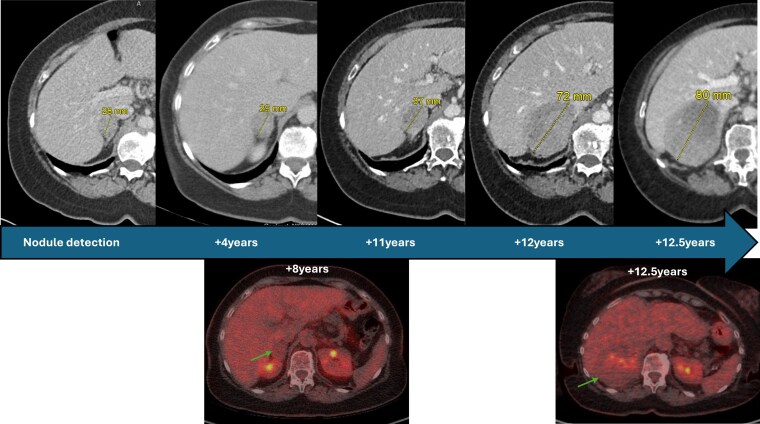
A timeline of portal phase intravenous contrast CT scans performed for case 2 demonstrating size stability of the right adrenal lesion for 11 years, followed by an accelerated growth trajectory. The bottom row shows 2 fused axial FDG-PET CT images demonstrating the non-FDG-avid homogenous right adrenal nodule at 8 years after detection, followed by a large right adrenal lesion with a heterogeneous pattern of FDG avidity at 12.5 years after detection (green arrows).
